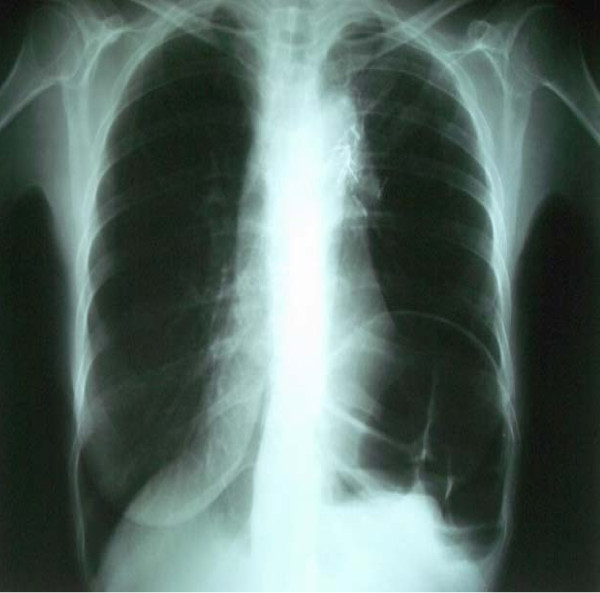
Chest radiograph taken on the day of the thrombus diagnosis.
